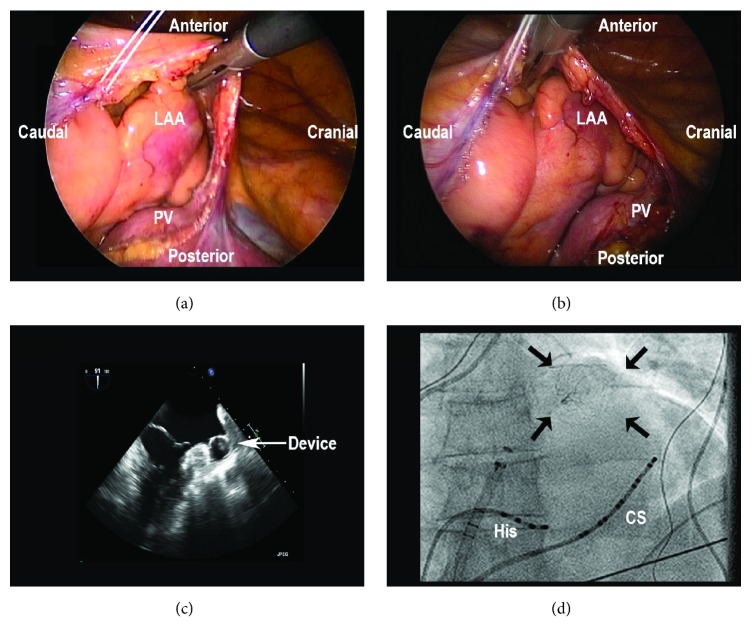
Watchman device implantation - Intraoperative images*. (a) Left-sided thoracoscopic view of the left atrial appendage (LAA) before implantation. PV = Pulmonary vein. (b) Left-sided thoracoscopic view of the LAA after implantation, showing that implantation of the device does not lead to significant morphological changes of the LAA. (c) Per-procedural echocardiographic view after implantation. (d) Per-procedural thoracic radiographic image after implantation. His = His Bundle catheter, CS = Coronary Sinus catheter.
